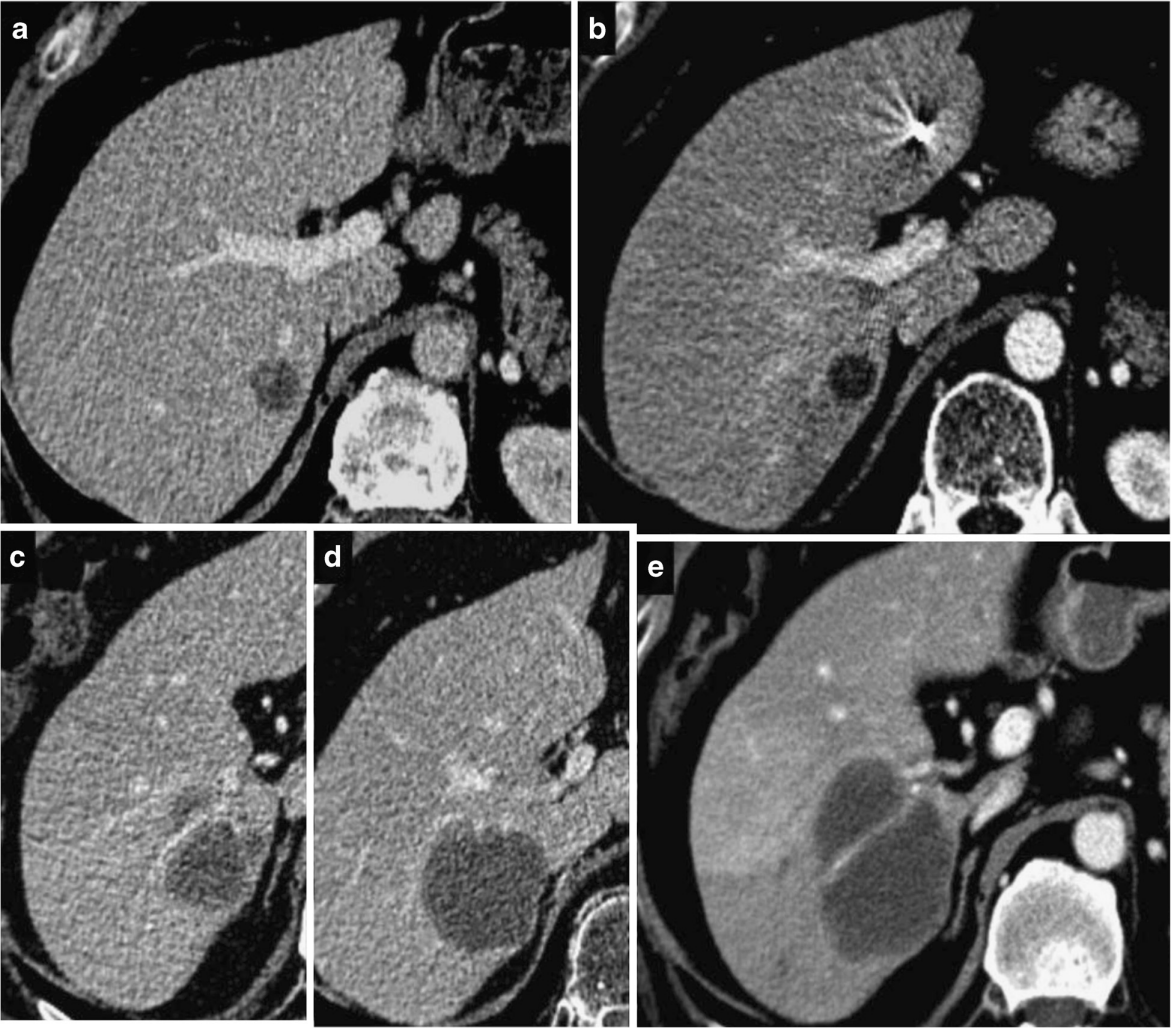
Pseudo- progressive lesion after SBRT, before treatment (a), 2 months later (b), 6 months later (c, d), and 9 months later (e). CT slices show the progressive size increase with a completely necrotic lesion.
